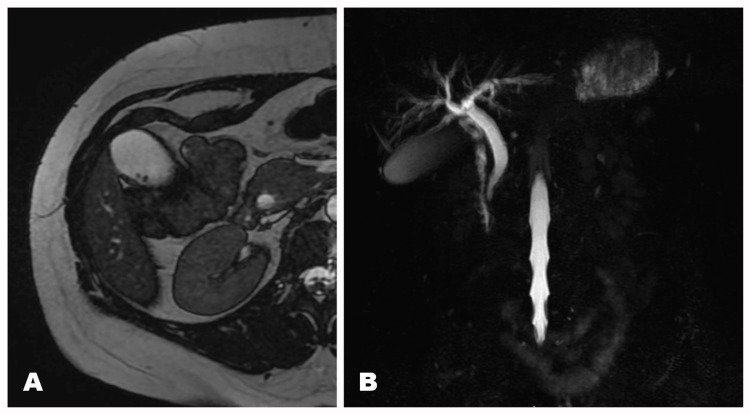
Abdominal MRI - obstructive jaundice of the common bile duct with multiple cholesterol gallbladder stones (A) T2-weighted axial MRI showing multiple infracentimetric gallstones within the gallbladder; (B) magnetic resonance cholangiopancreatography (MRCP) revealing distal segment obstruction of the common bile duct.
